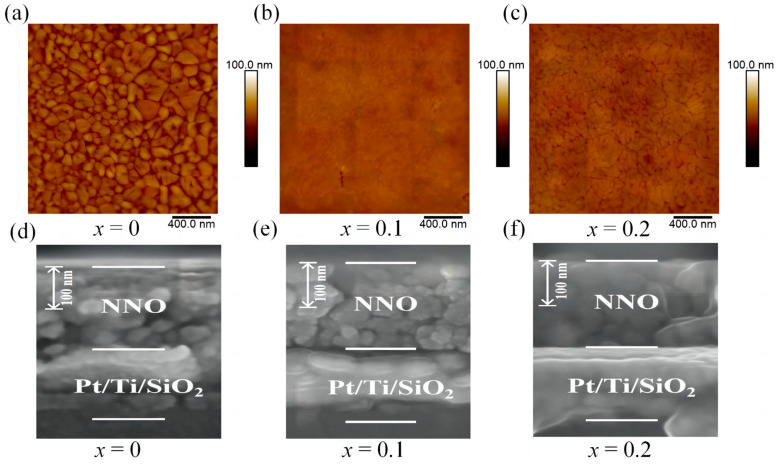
AFM and cross-SEM images of (1−*x*)NN-*x*CT thin films. (a,d) *x* = 0, (b,e) *x* = 0.1, (c,f) *x* = 0.2.
